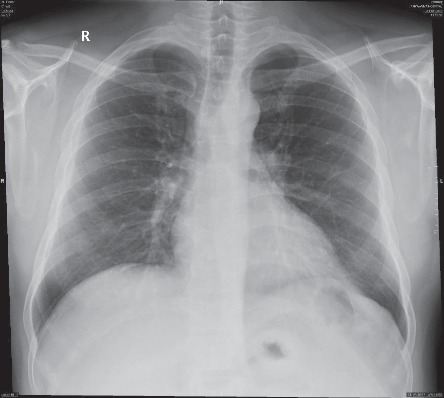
Preoperative chest radiograph for the patient showing normal lung fields with mild increase in bronchocovascular markings on the right lung.
